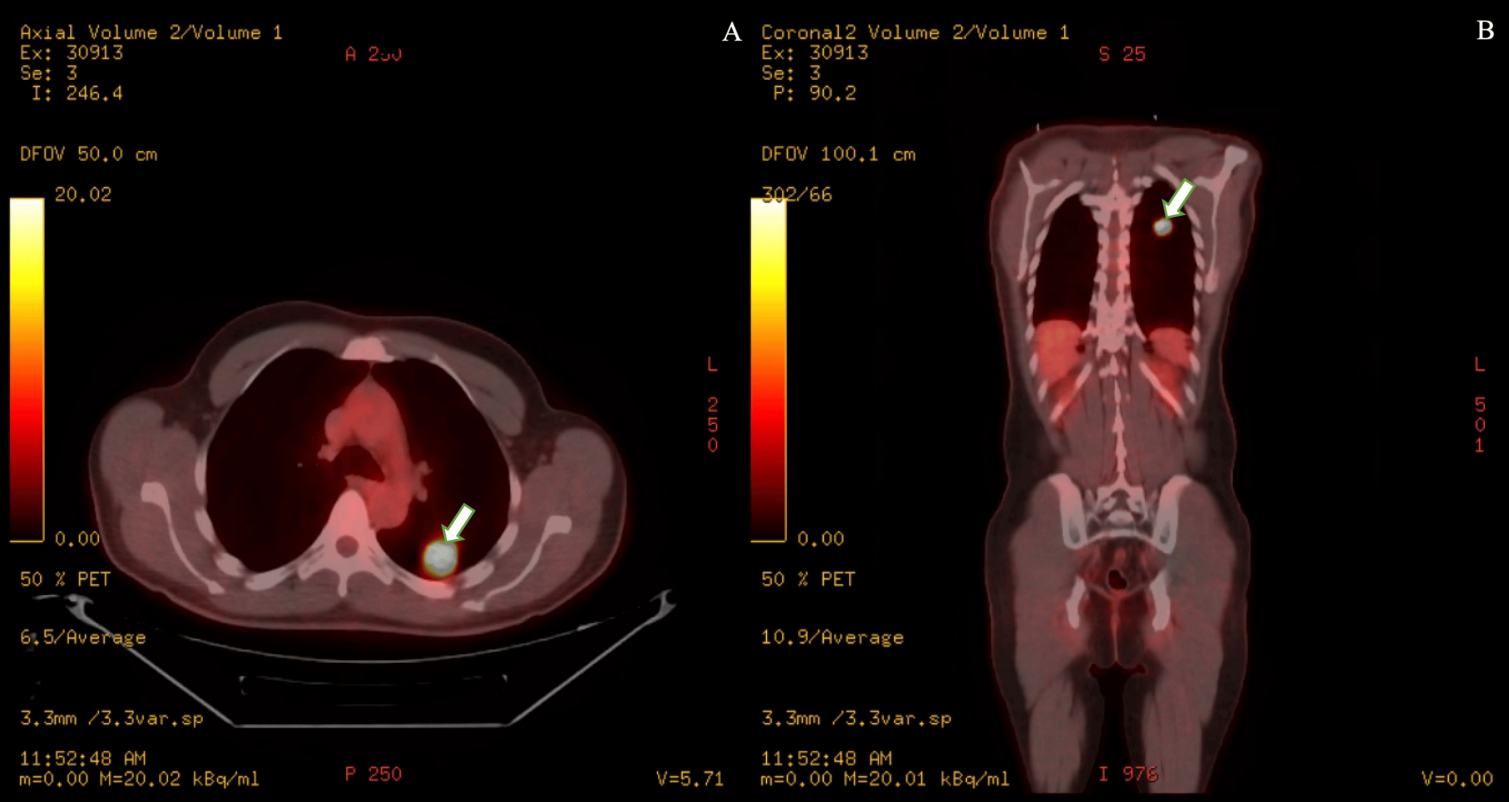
Hyperbolic left lower lobe lung nodule with a maximum SUV of 17.2; A) axial view, B) coronal view. SUV, standardized uptake value
